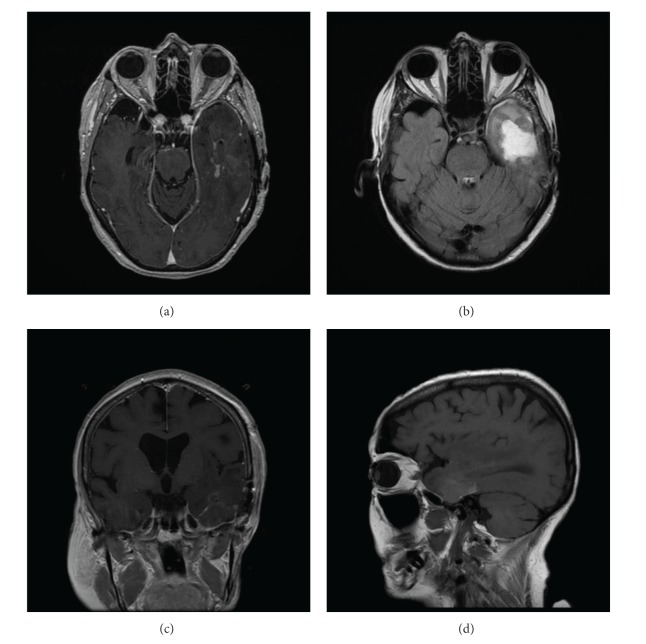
Axial T1 postcontrast (a), T2 FLAIR (b), coronal T1 postcontrast (c), and sagittal T1 postcontrast (d) MRIs obtained on postoperative day 1 demonstrated total resection of the enhancing mass seen on preoperative imaging. There were some minor blood products and enhancement, consistent with expected normal reactive change after surgery.
